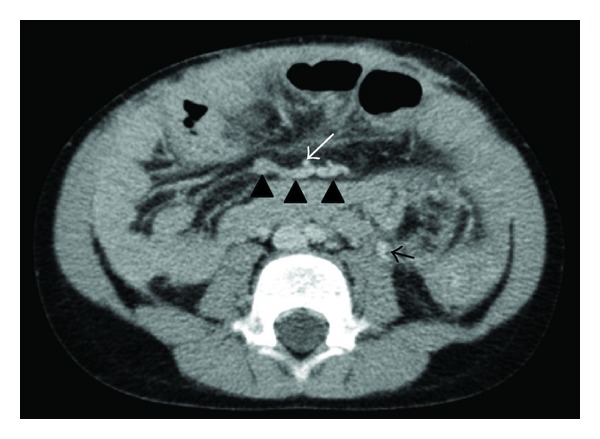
Post IV contrast transverse axial CT scan: SMA (white arrow), no normal SMV demonstrated, confluence of collateral veins (black arrow heads), large inferior mesenteric vein that drains to the splenic vein (black solid arrow).
